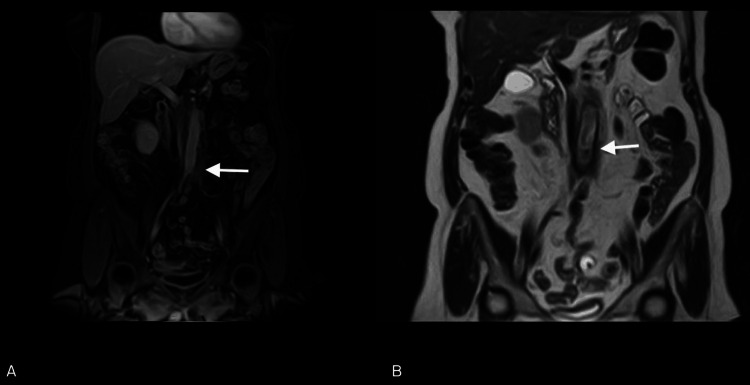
MRI abdomen with contrast enhancement: periaortic thickness (arrow in A) with sign of inflammation/activity (arrow in B). Thickening of the adipose tissue in the peri-aortic area, forming a "cuff," with a maximum sub-centimeter thickness, extending along the sub-renal abdominal aorta to the carrefour, involving the root of the left common iliac artery. Signal characteristics are low on T1-weighted images (A) and intermediate to high on T2-weighted images (B), consistent with inflammatory/active tissue characteristics. Millimetric lymph node formations are present in the common iliac area, showing restricted diffusivity.
